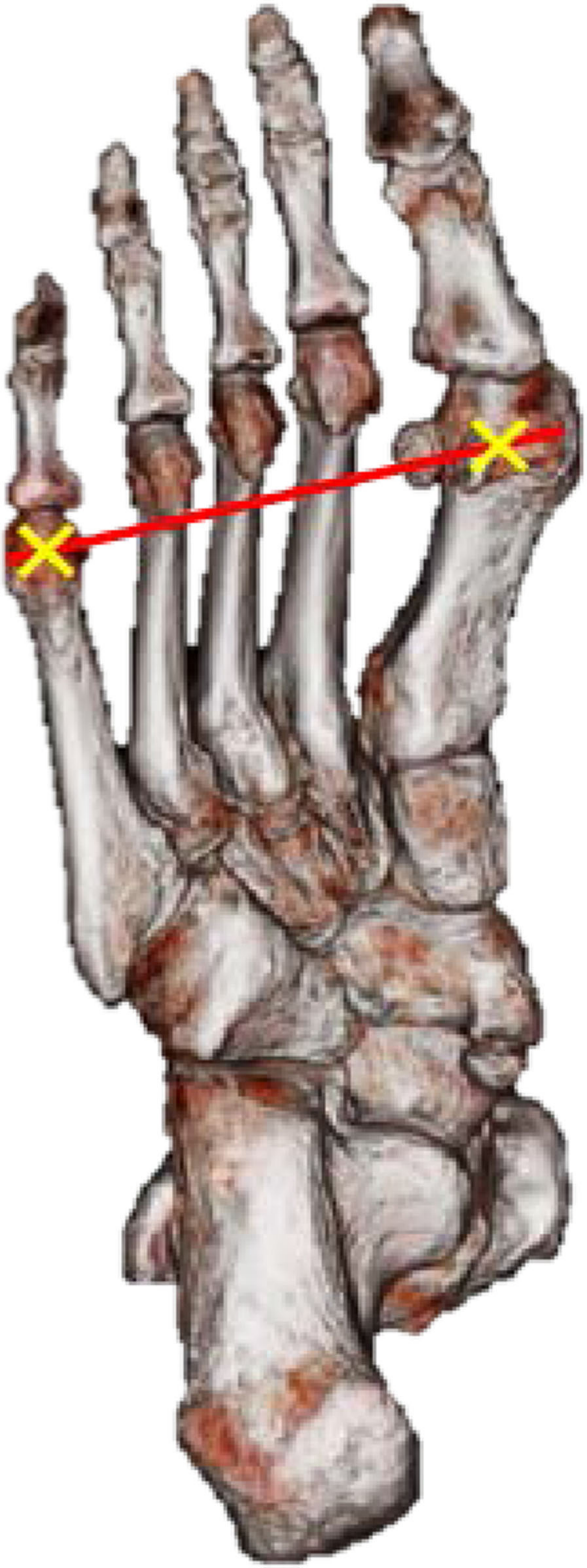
Landmarks for ultrasonography (US) and computed tomography (CT). The 1st metatarsal head (MTH), which includes the sesamoid bones and 5thMTH, were the landmarks for US and CT scans. For US, the probe was located over the line (*red line*) through both landmarks (*yellow marks*). For CT, the image with both landmarks was selected
